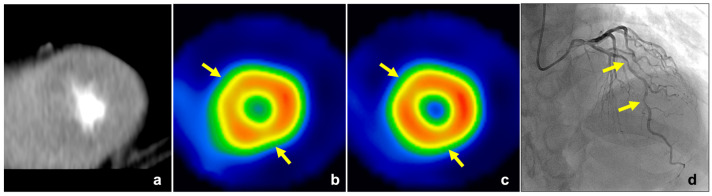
A 64-year-old man with effort angina. No obvious low-attenuation area was observed in the stress CTP image for the visual assessment (a), but the perfusion abnormalities were detected with MPR in the anterior and inferior myocardium of the apex (MPR: 0.69 and 0.71 < cut-off value: 0.81, respectively). SPECT ((b), stress; (c), rest) showed reversible perfusion abnormalities in the anterior and inferior myocardium in the apex (yellow arrow). ICA revealed tandem lesions with moderate and severe stenosis in the LAD (yellow arrow, (d)). The FFR of LAD was 0.68. CTP, computed tomography perfusion; MPR, myocardial perfusion ratio; SPECT, single-photon emission computed tomography; ICA, invasive coronary angiography; LAD, left anterior descending artery; FFR, fractional flow reserve.
